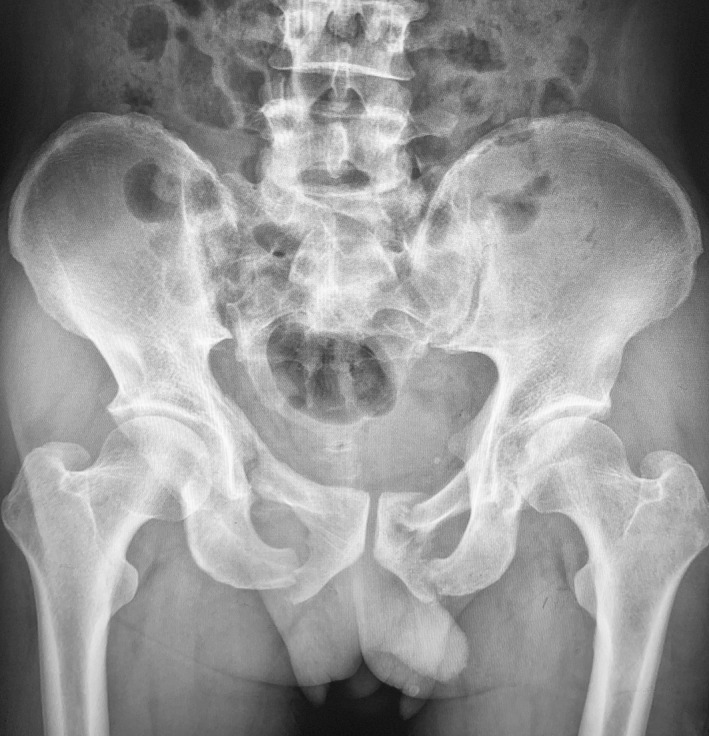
A 57‐year old male patients with LE‐PFs. Pelvic radiograph showed fractures on the left superior and inferior pubic ramus and right inferior pubic ramus
